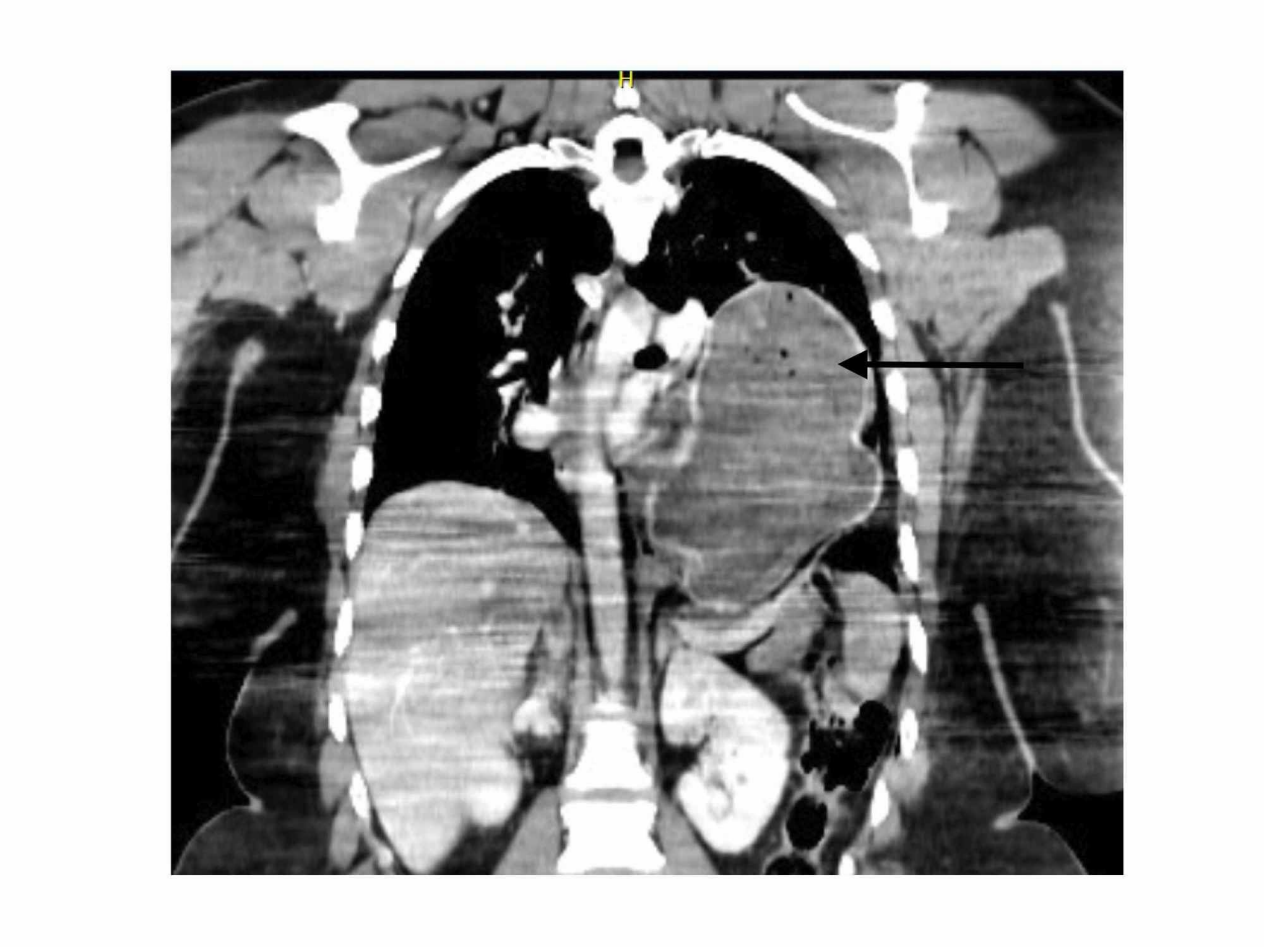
CT section showing herniation of stomach in left hemi-thorax
